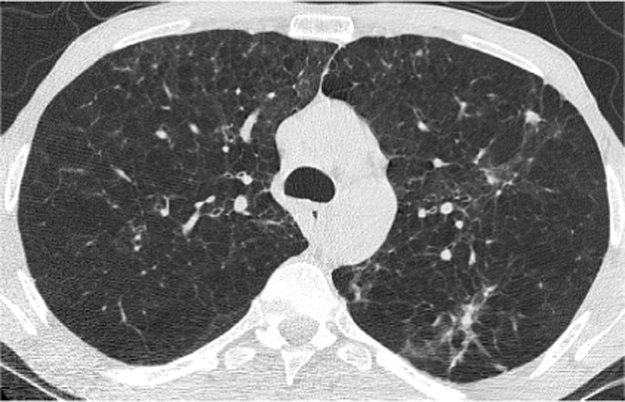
CT thorax from December 2015 demonstrating multiple pulmonary nodules.
